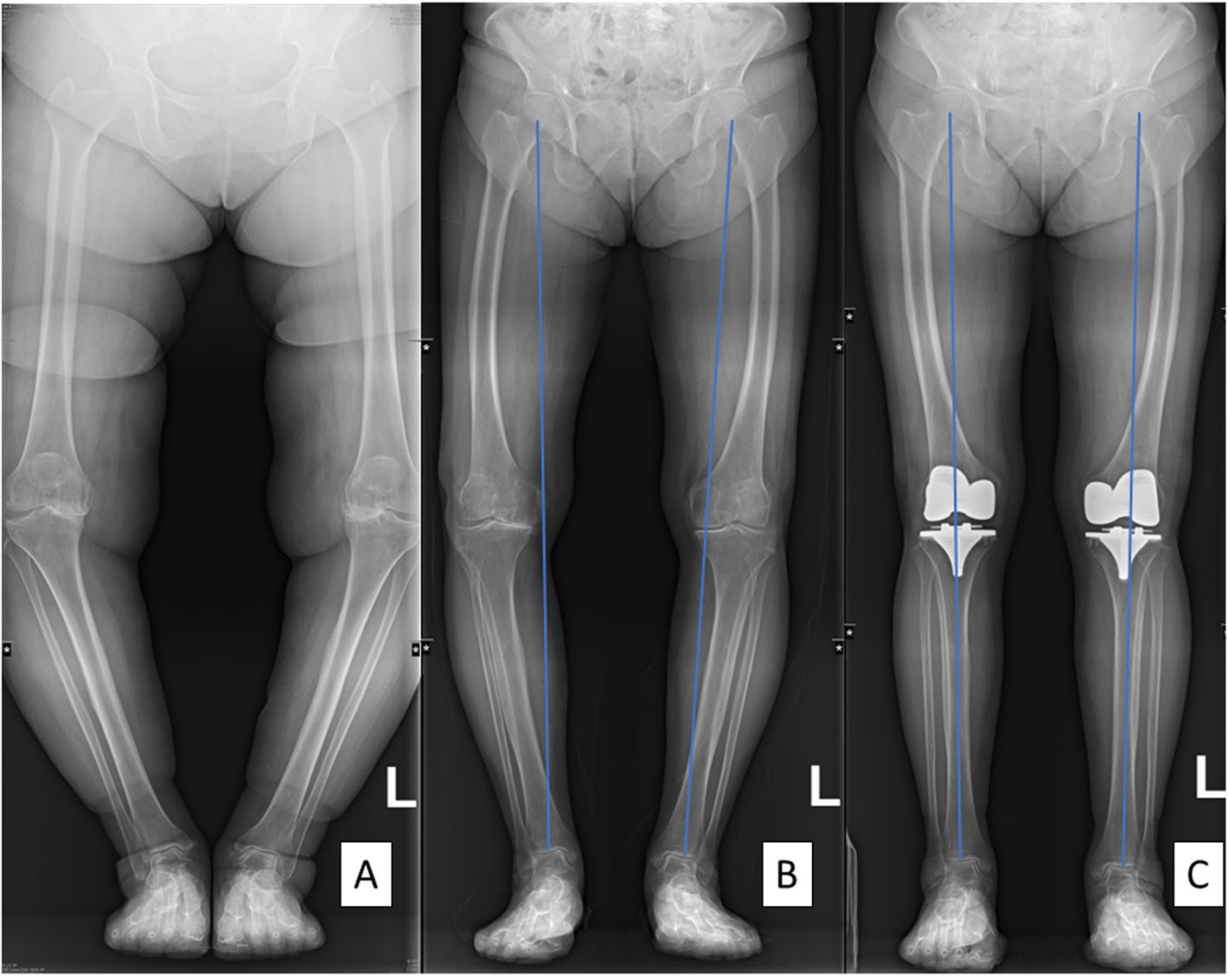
Lower limb long-film X-ray. Severe genu varum in a 57-year-old female patient with no extraarticular deformity (a). Bilateral severe femoral bowing seen preoperatively in an 85-year-old female patient enrolled in this study (b). Postoperative X-rays of patient B (c). The right side was treated using conventional instruments and the left side was treated using PSI
